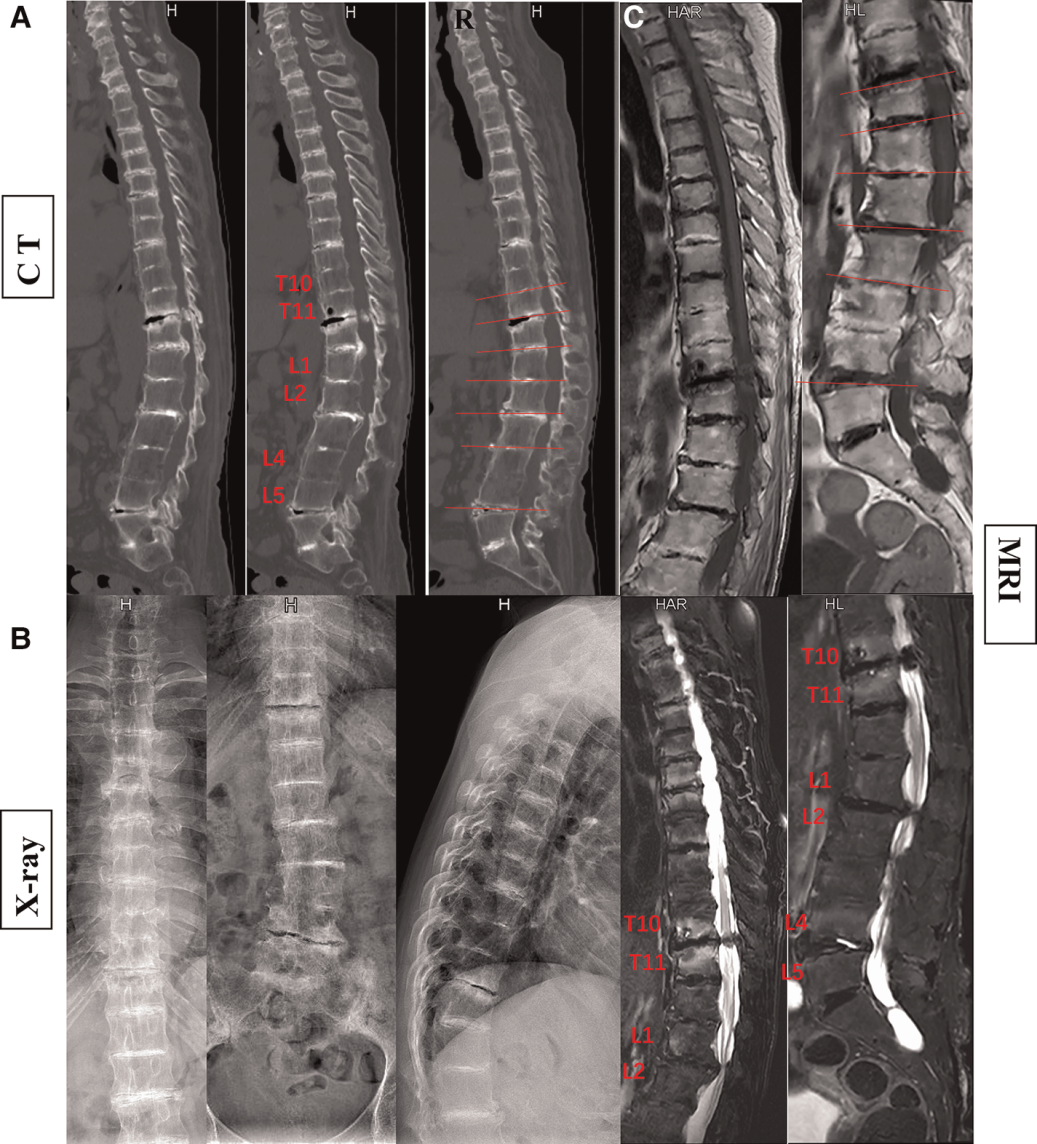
The patient's preoperative imaging examination revealed kyphosis and spinal stenosis. Disruption and stenosis of the intervertebral disc with labral changes. Intervertebral ossification dysplasia, vertebral body without ossification connected (A, CT; B, x-ray; C, MRI).
